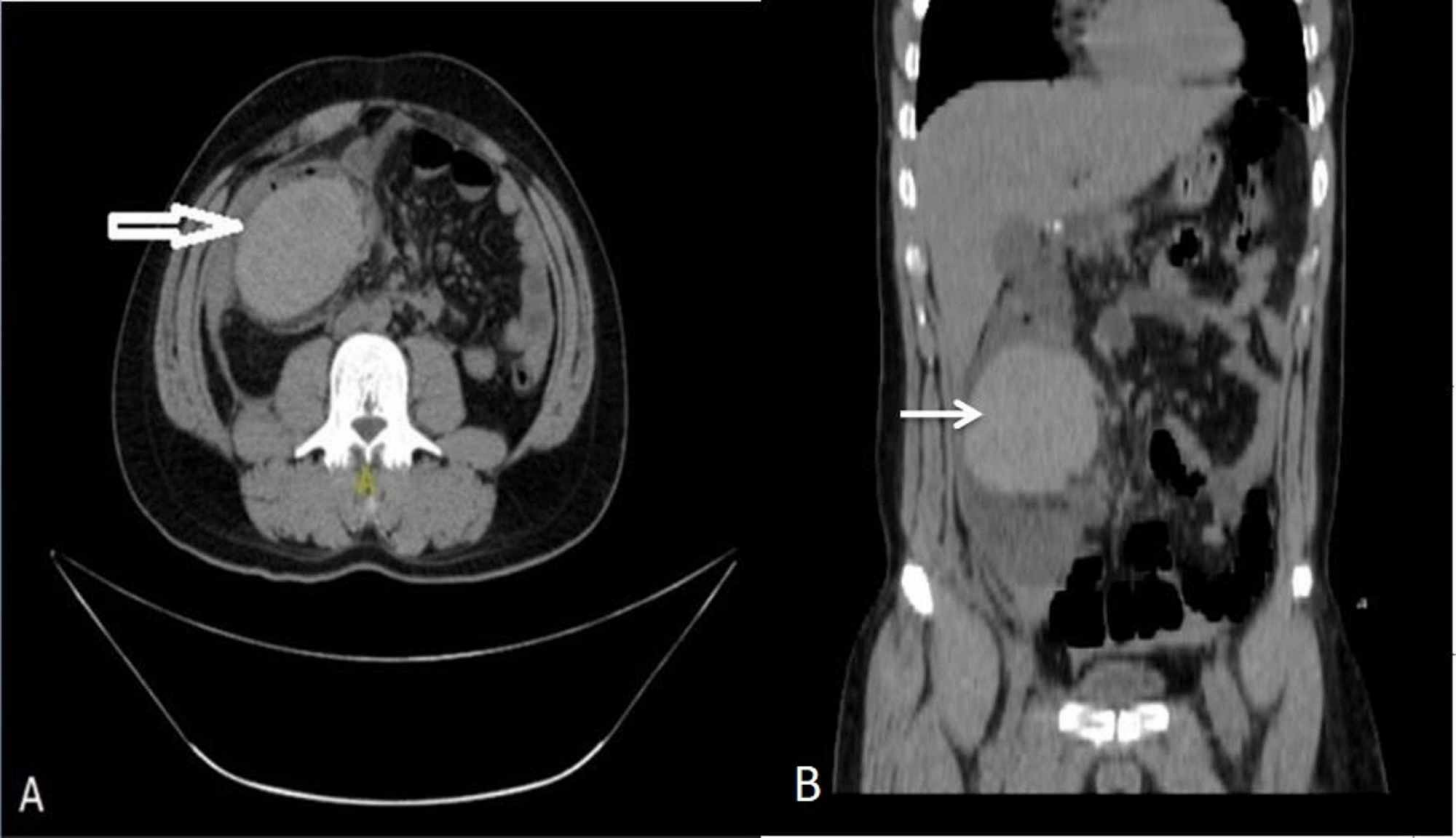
Contrast-enhanced computed tomography (CECT) abdomen showing intramural hematoma (arrow) of ascending colon. (A) Coronal view and (B) sagittal view.
